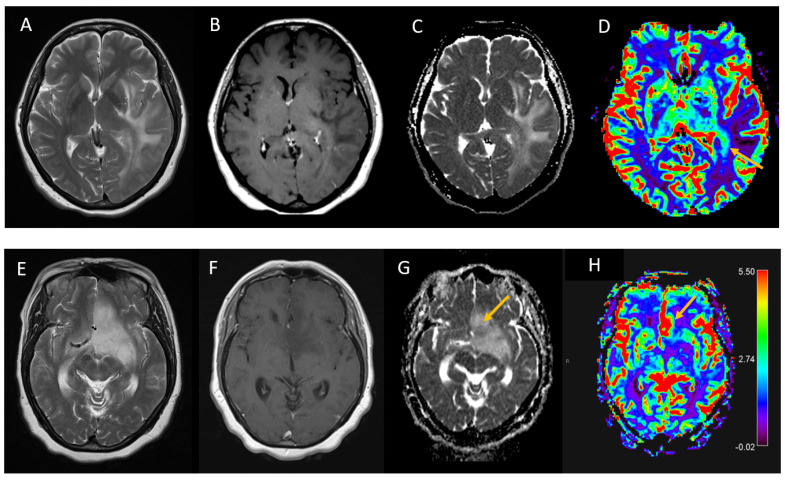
T2-weighted and gadolinium-enhanced T1-weighted images, ADC maps and dynamic susceptibility contrast-enhanced (DSC) imaging-derived rCBV maps in two individuals with WHO grade 4 IDH wild-type glioblastoma (A–D) and WHO grade 3 IDH1 mutant diffuse astrocytoma (E–H) showing elevated tumour perfusion (arrows).
